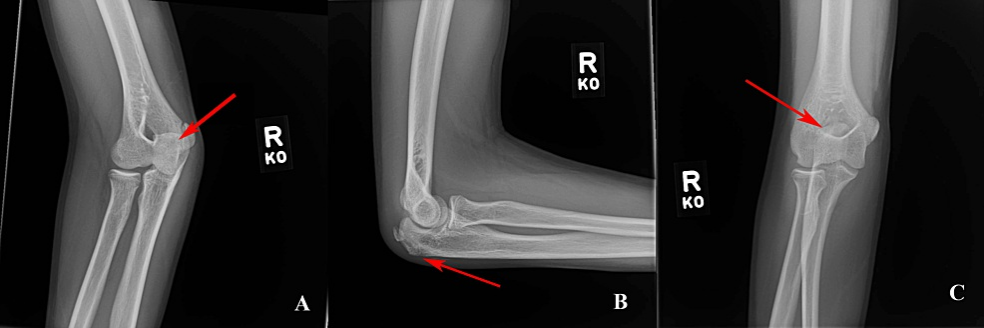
AP (A), lateral (B), and oblique (C) radiographs of the right elbow demonstrating an avulsion fracture of the olecranon. AP: anterior-posterior.
